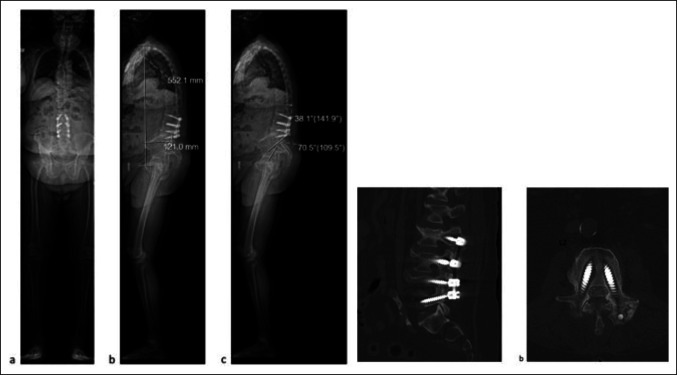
Left: (A) Preoperative AP and (B) preoperative lateral EOS standing radiographs demonstrating sagittal imbalance with a positive sagittal vertical axis of 12.1 cm. C, Preoperative radiograph showing pelvic incidence measured at approximately 70° with a lumbar spine lordosis of approximately 38°. Right: (A) Sagittal and (B) axial lumbar CT scan demonstrating pseudarthrosis with large halos around the previously placed pedicle screws.
